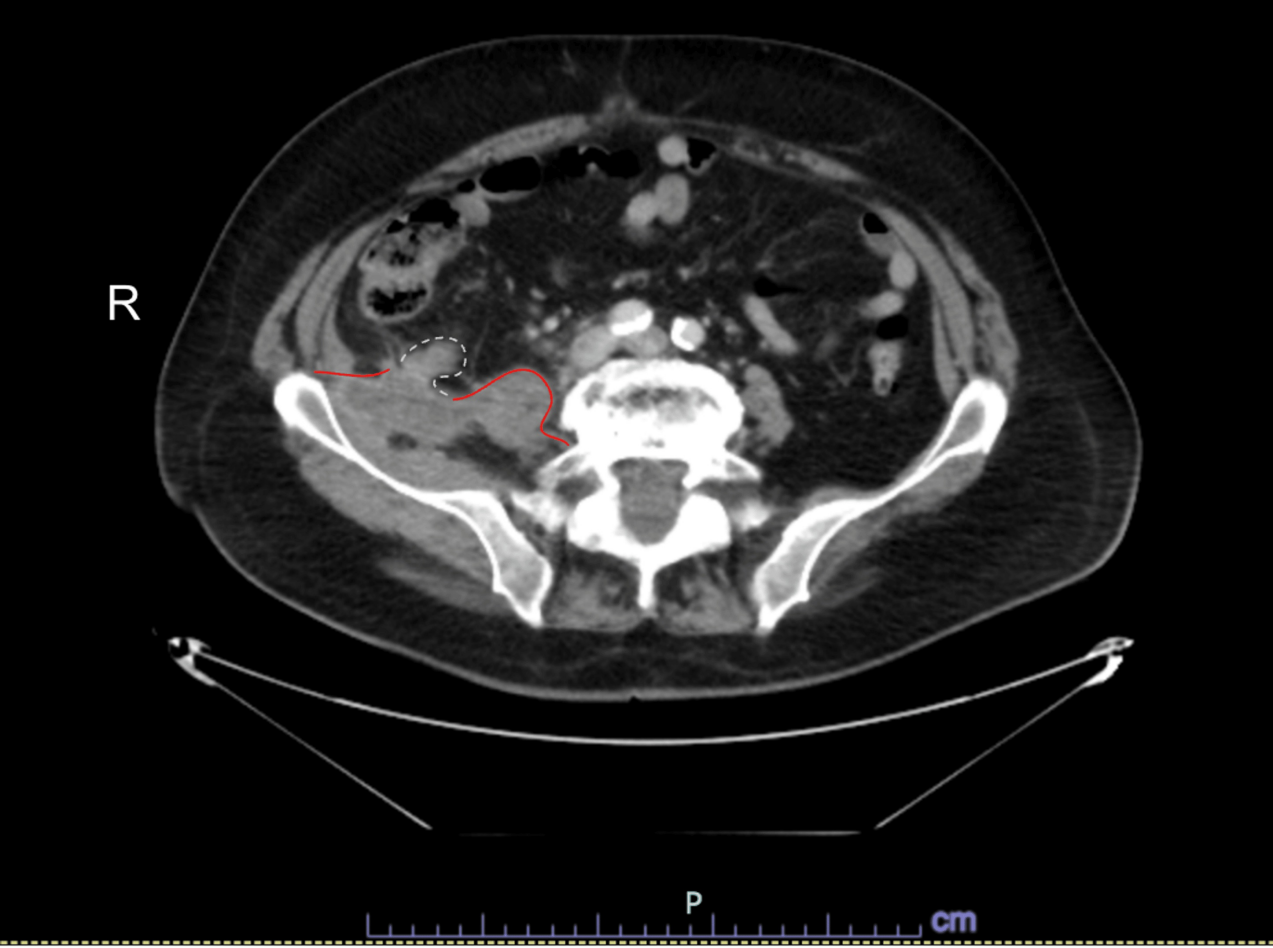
Transverse view CT depicting the appendix fistulized to the iliopsoas accompanied by abscess formation. White dotted line: border of the appendix. Red line: border of the iliopsoas.
